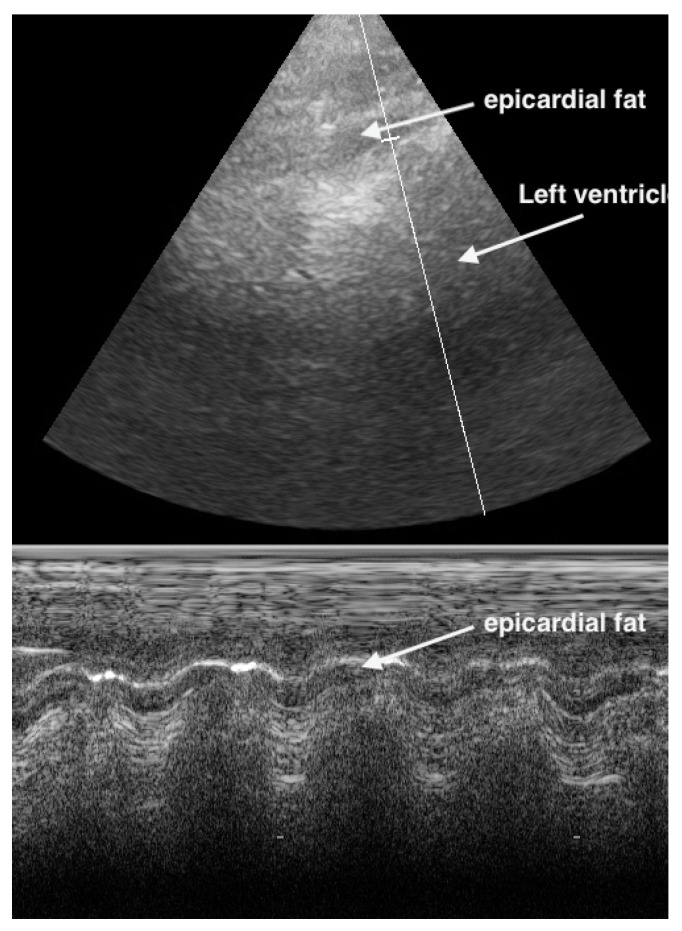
In the apical 4CV (Chambers almost not to see), in the apex is a broad hypoechoic band which has always the same size. 4CV = Four Chamber view.
